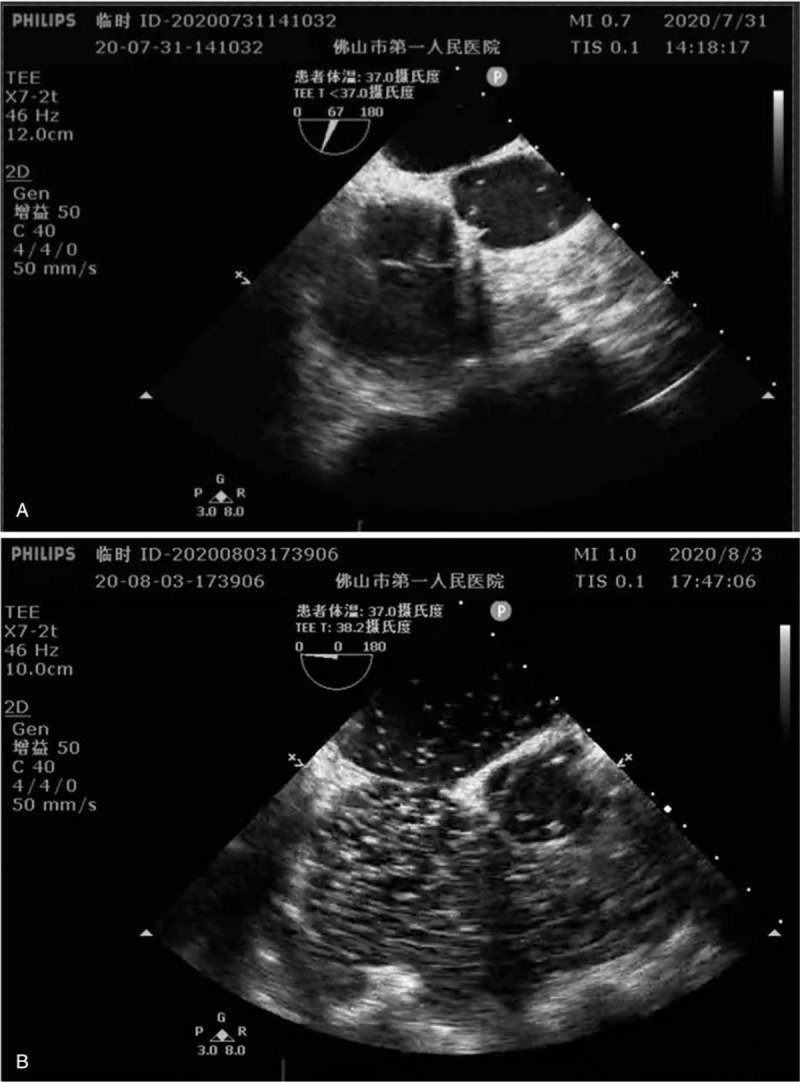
Transesophageal echocardiography demonstrated air within heart chambers.
